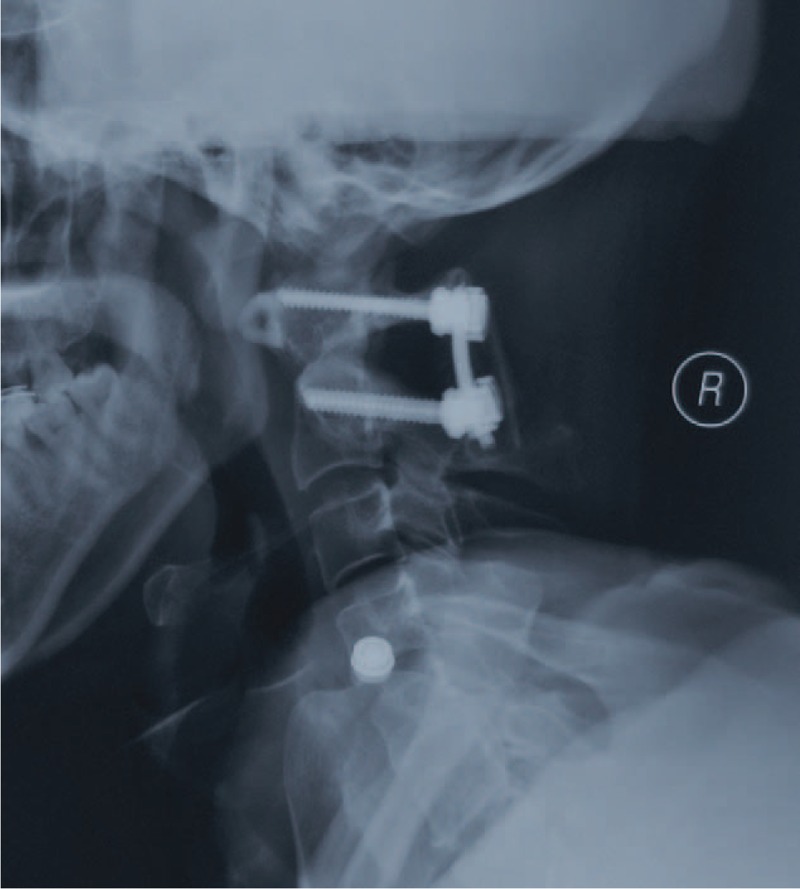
Postoperative lateral radiography showed anatomic alignment of the atlantoaxial complex with posterior polyaxial screw-rod fixation.
